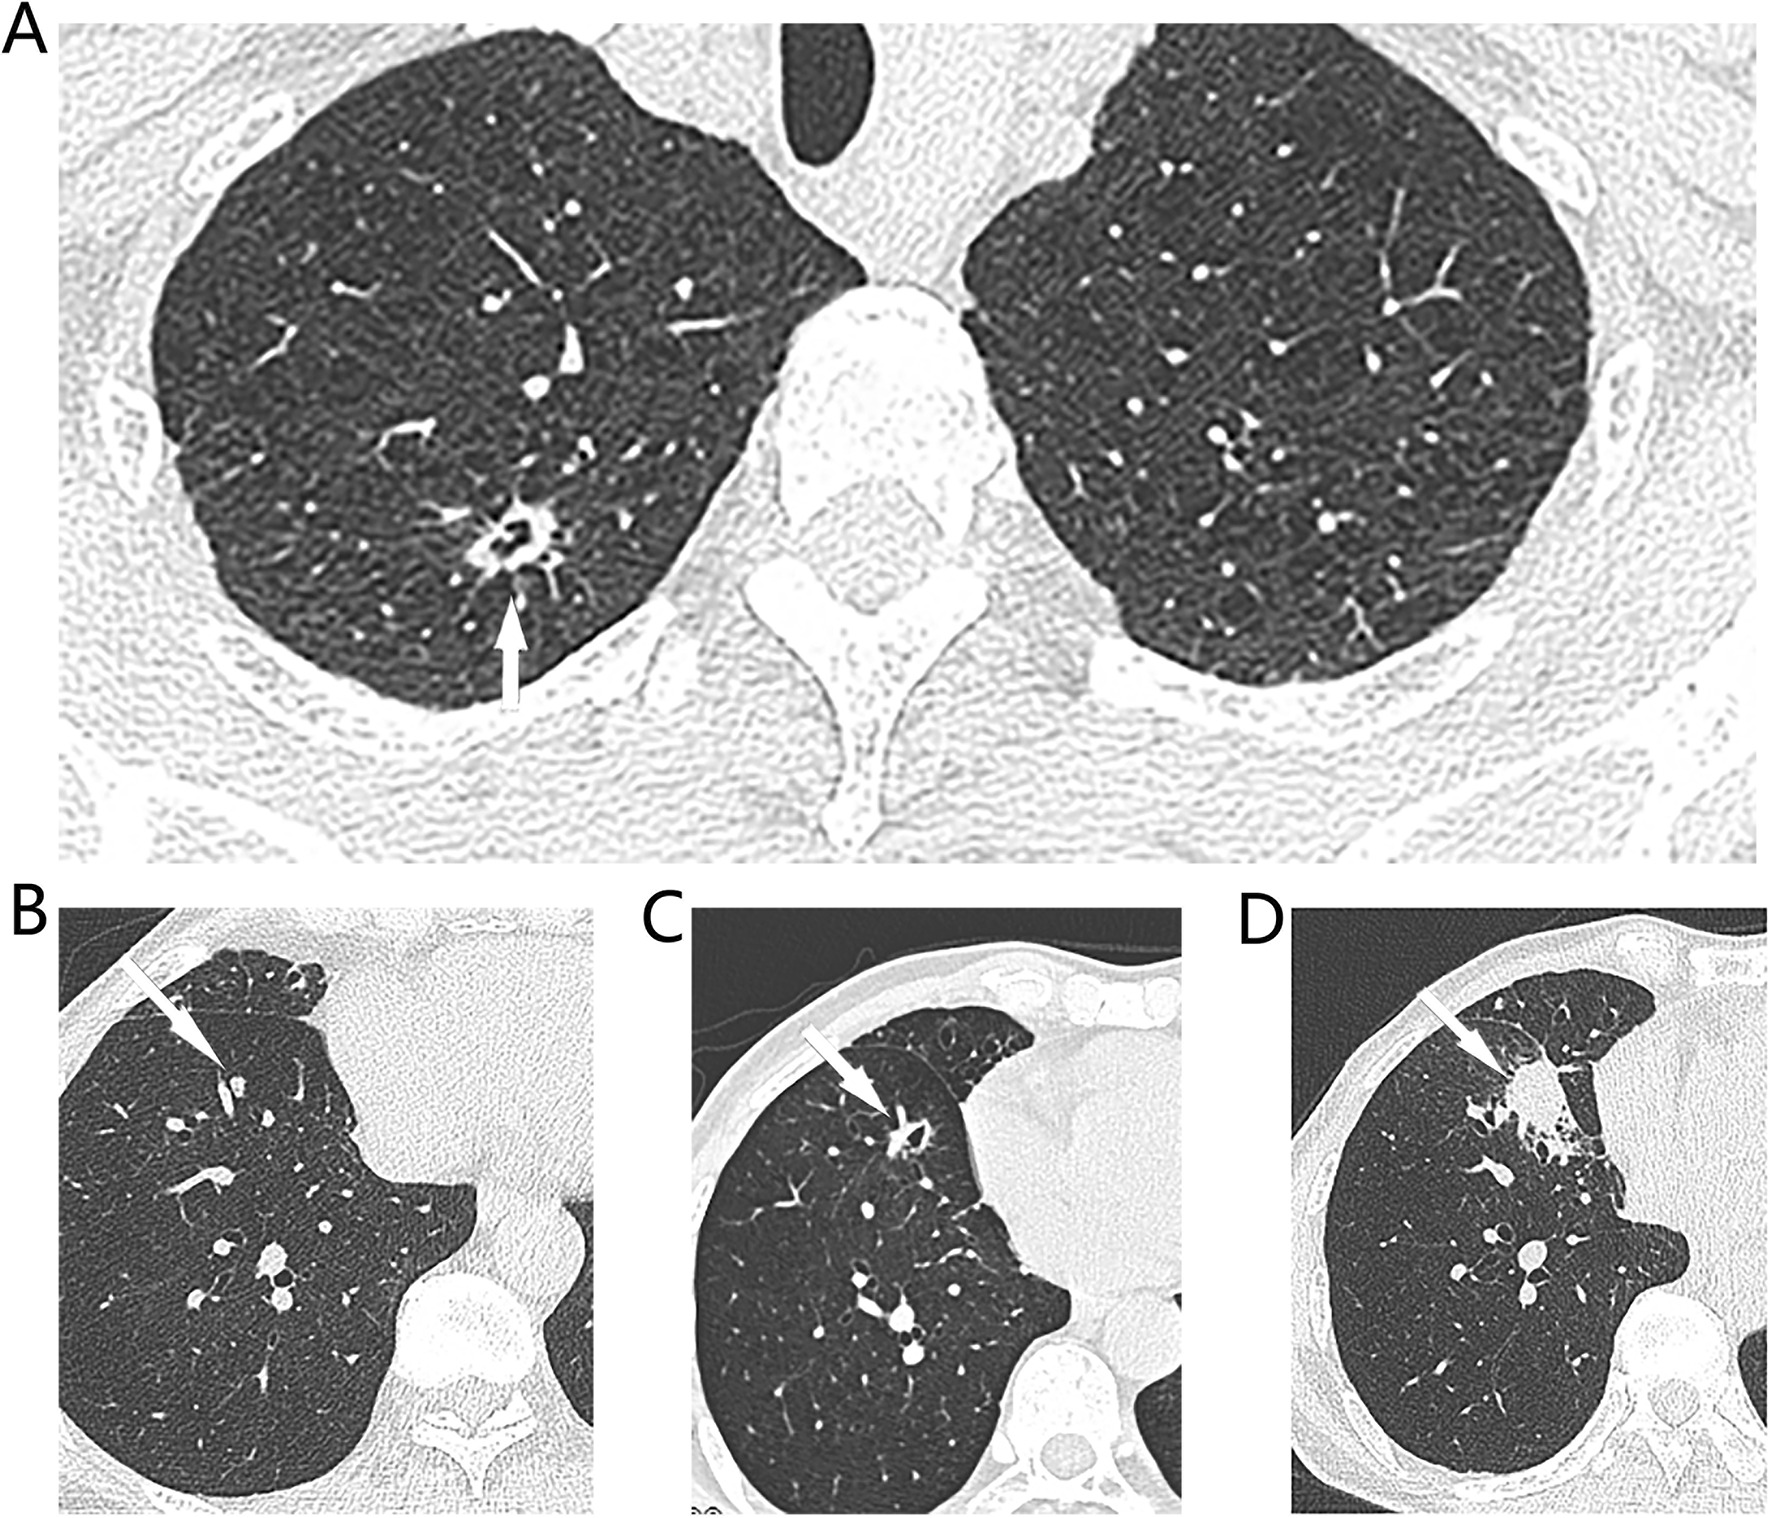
Typical CT images of synchronous multiple primary lung cancers. A On preoperative CT of a 67-year-old male patient shown a 13*8 mm cystic nodule (white arrow) in the right upper lobe, and B a 5 mm solid nodule (white arrow) in the S8 segment of right lower lobe. Patient received right upper lobectomy and systemic lymph node dissection in July 2019 and confirmed as adenocarcinomas (80% acinar adenocarcinoma, 10% solid adenocarcinoma) on surgical pathology, pT1bN0M0, IA2. The small solid indetermined nodule of the S8 segment of right lower lobe was followed up. C 5 months later, the nodule had grown up to a 10 mm cystic nodule. D 14 months later, this nodule had grown up to a 24*20 mm solid nodule and with multiple N2 lymph node enlargement, CT guided core needle biopsy shown acinar predominant adenocarninoma. This patient was diagnosed with synchronous multiple primary lung cancers. Because of multiple N2 lymph node metastasis and no driver gene mutation, he received Anti-PD-1 Therapy plus Chemotherapy
